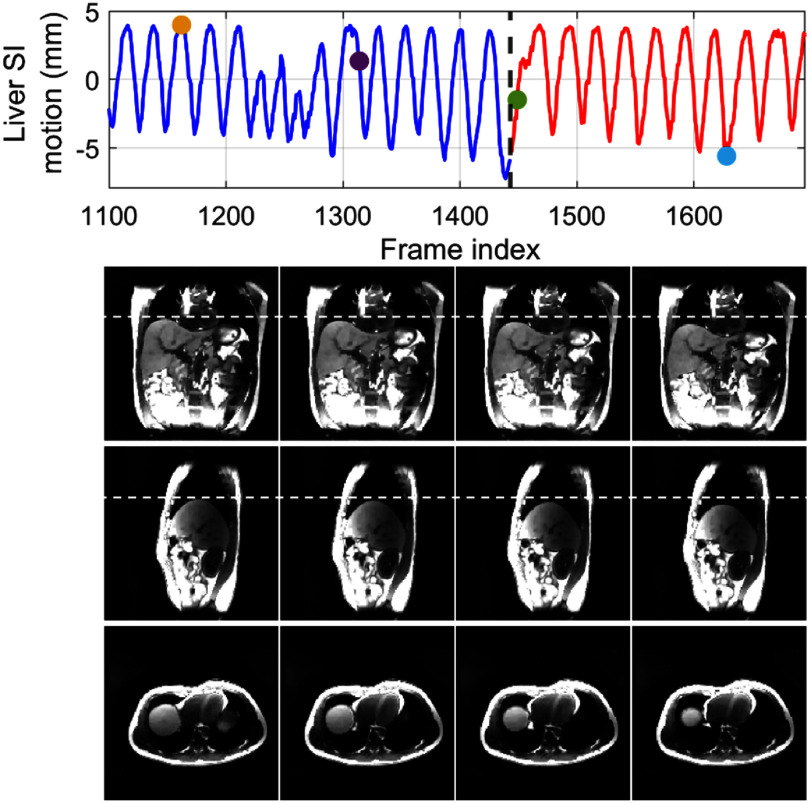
Dynamic MR images of the human subject study. The first row shows the estimated liver center-of-mass trajectory along the SI direction, with dots indicating the time points selected for plotting. The vertical dashed line at frame 1443 shows the separation between the dynamic reconstruction and real-time imaging tasks. The subsequent rows display the selected MR images in the coronal, sagittal, and axial views. The first two columns correspond to the dynamic reconstruction task, while the last two columns correspond to the real-time imaging task.
